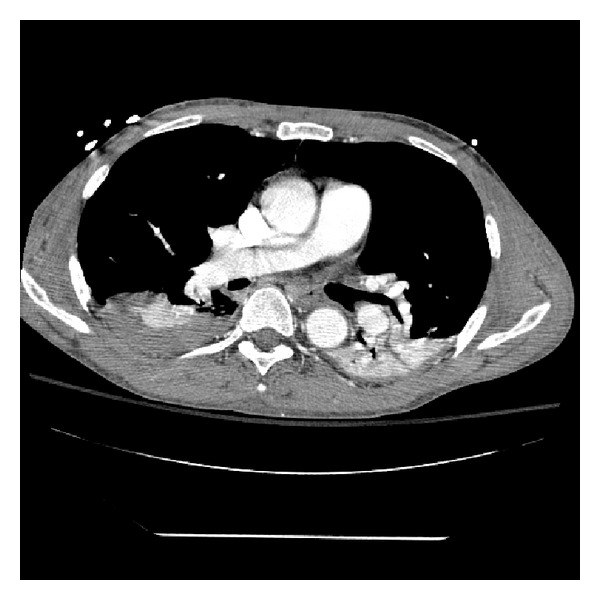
CT angiogram of the chest after thrombolysis and anticoagulation with resolution of visible thrombi.
